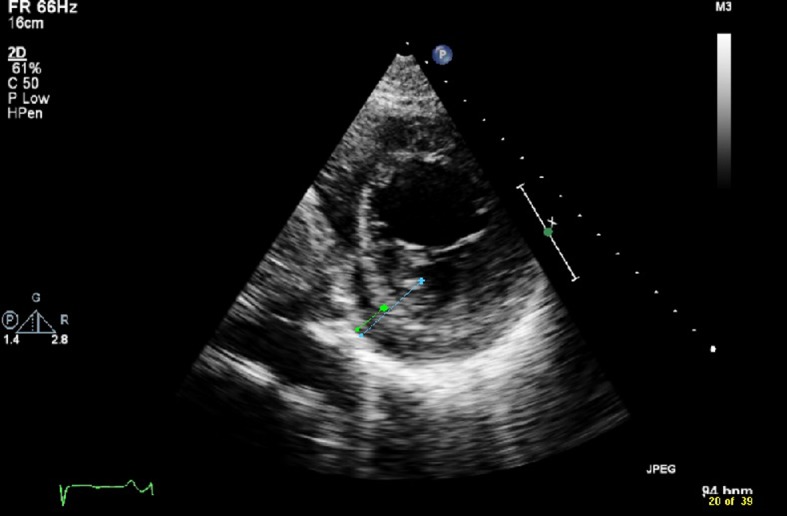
Transthoracic echocardiogram with short parasternal view shows prominent trabecular projections with the distance from the epicardial surface to the trough of the trabecular recess X (green line) of 1.3 cm, and with the distance from the epicardial surface to the peak of trabeculation Y (blue line) of 3 cm with ratio X/Y < 0.5.
